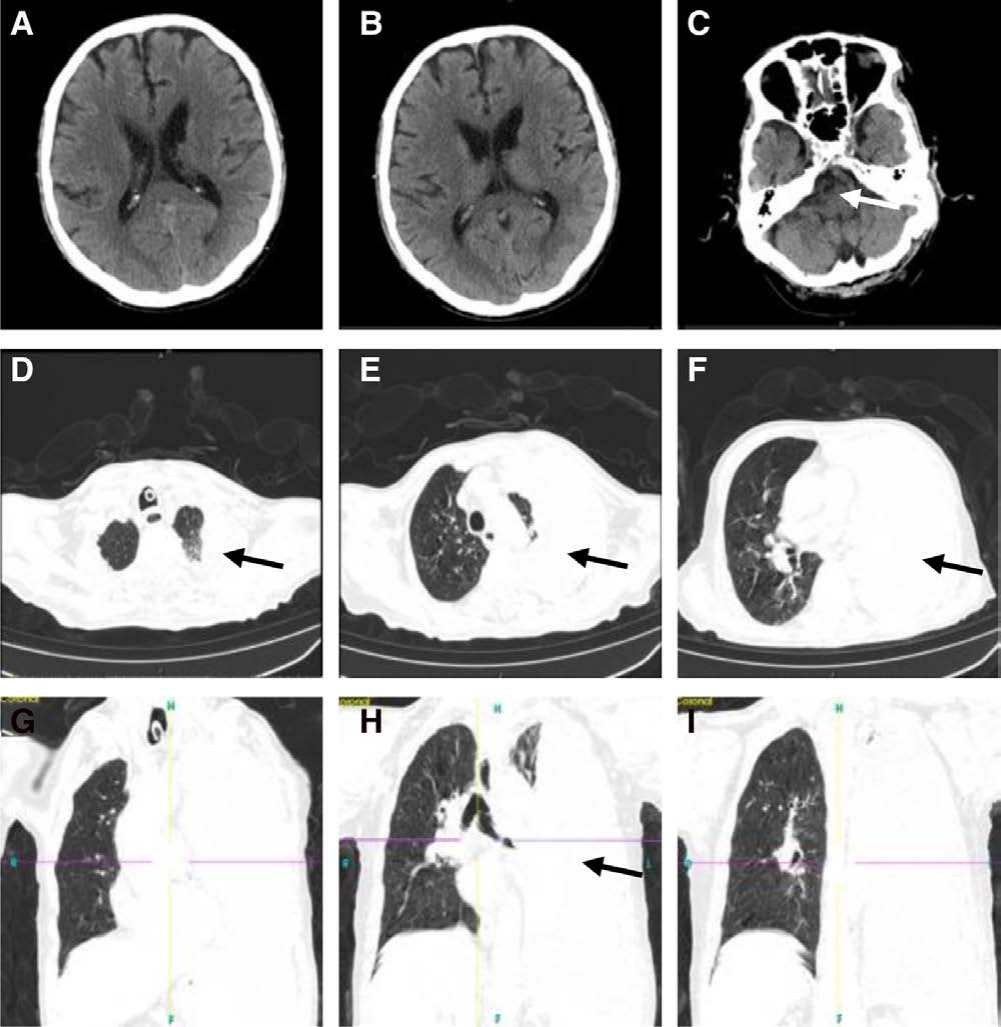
Cranial and thoracic CT scans after admission. Cranial CT (A–C) revealed cerebral infarction in the basal ganglia and the brainstem, along with a right hemioval center softening lesion, causing hemiplegia, dysphagia, and pulmonary dysfunction; thoracic CT (D–I) showed left pleural effusion, causing dyspnea. CT = computed tomography, PD = pulmonary dysfunction.
